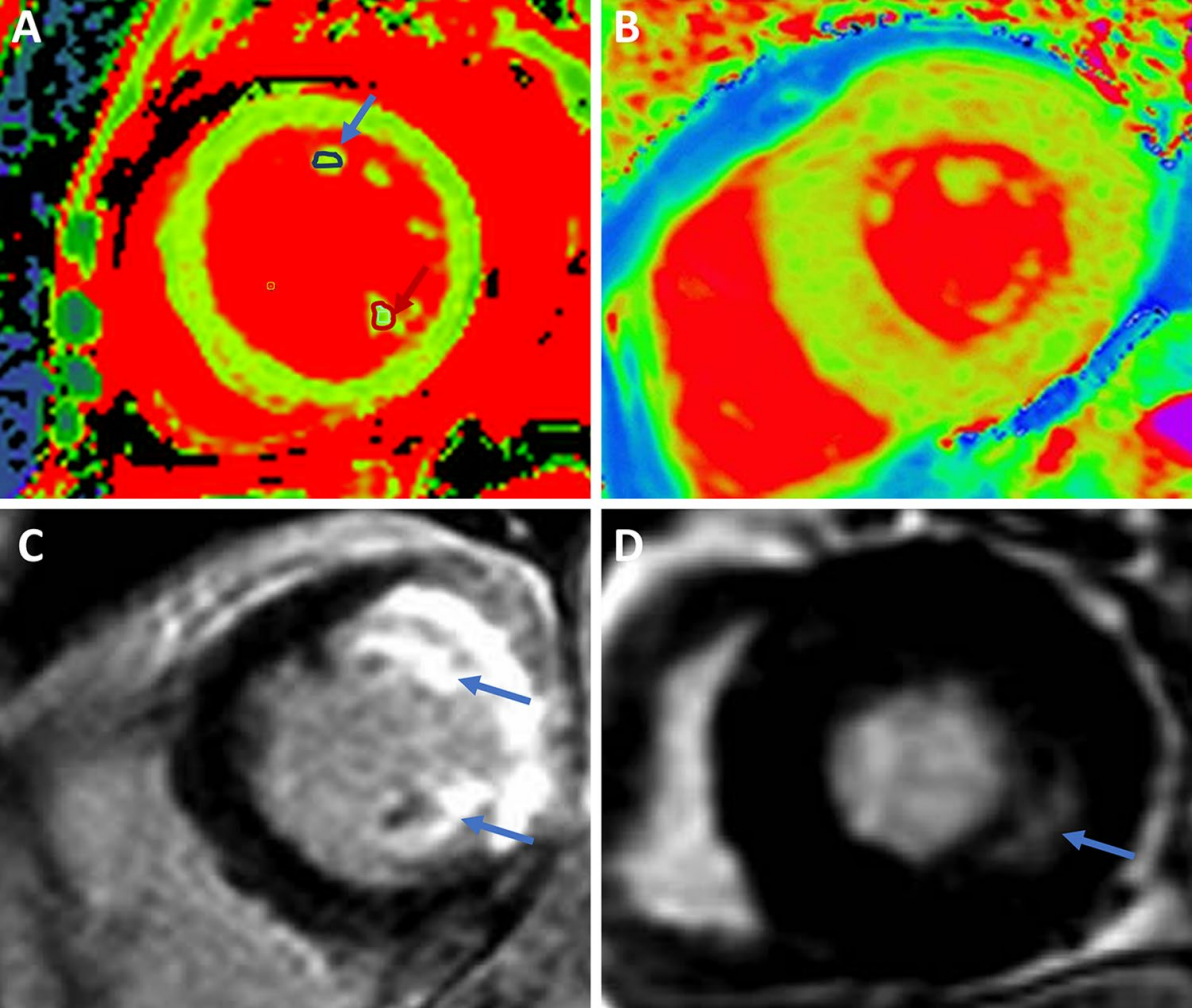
Tissue characterization with cardiac magnetic resonance imaging. The figure shows four examples of patients with valvular heart disease and the use of cardiac magnetic resonance imaging to assess left ventricular myocardial fibrosis. Patient 1: a 44-year-old female with prior myocarditis and a left ventricular ejection fraction of 21% presented with severe secondary mitral regurgitation. Regions of interest for native T1 time have been drawn inside both papillary muscles (blue and red arrow) and show an elevated native T1 time of the anterolateral (1184 ms) and the posteromedial papillary muscles (1148 ms) (panel A). Patient 2: a 78-year-old male patient presented with severe aortic stenosis and preserved left ventricular ejection fraction of 58%. The T1 time of the left ventricular myocardium is increased (984 ms) (panel B). Patient 3: a 54-year-old male patient presented with a lateral myocardial infarction and necrosis of both papillary muscles (*blue arrows*), causing severe mitral regurgitation (panel C). Patient 4: a 76-year-old female patient presented with severe aortic stenosis. Cardiac magnetic resonance imaging shows the presence of mid-wall replacement fibrosis (*blue arrow*) (panel D)
